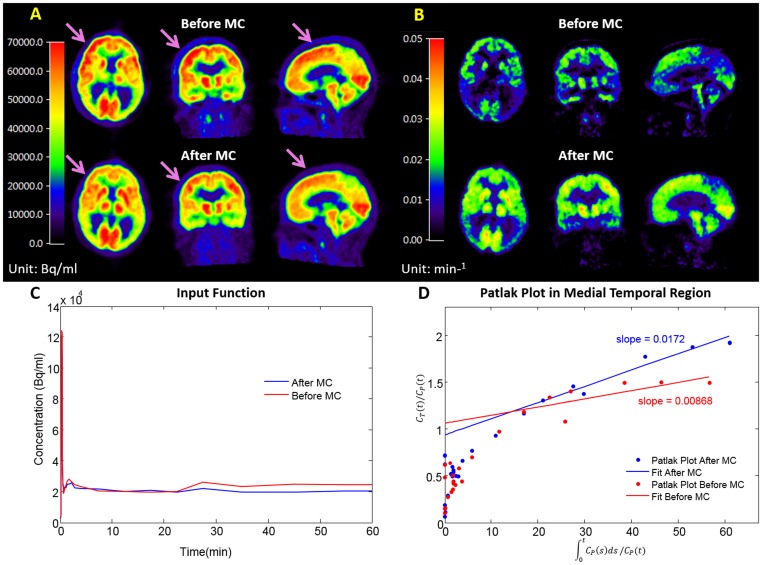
Improvement of a FDG PET/CT scan of a DS patient after MC in aspects of late frame, K_i_ image, image derived input function and Patlak Plot. A. Orthogonal PET/CT images before and after MC of a late frame in the dynamic brain FDG scan: attenuation correction artifact in the right frontal region (pointed at by arrows) diminished after MC (lower row). B. The K_i_ image: The K_i_ values appeared to be more uniform (except the affected regions) and globally higher after MC. In this case, the lower input function accounted for the global increase in the K_i_ values C. Image Derived Input Function: After MC, a 21% drop in the tail AUC during 30–65 minutes was seen. D. Patlak Plot in the medial temporal region before and after MC: The Patlak plot had less fluctuation after MC. The slope of the plot used for calculating K_i_ increased by 97.9% after MC for this patient.
